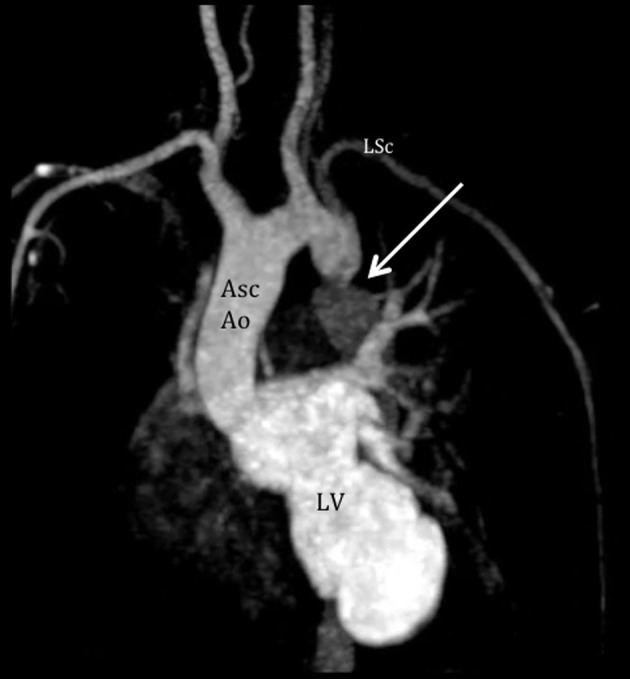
Cardiac MRI demonstrates the coarctation of the aorta. Aortogram shows severe juxtaductal coarctation of the aorta (arrow), 20 mm from the take off of the left subclavian artery (LSc). There is antegrade flow from the LV with significant compromise after the coarctation. Asc Ao: ascending aorta; LSc: left subclavian artery; LV: left ventricle.
